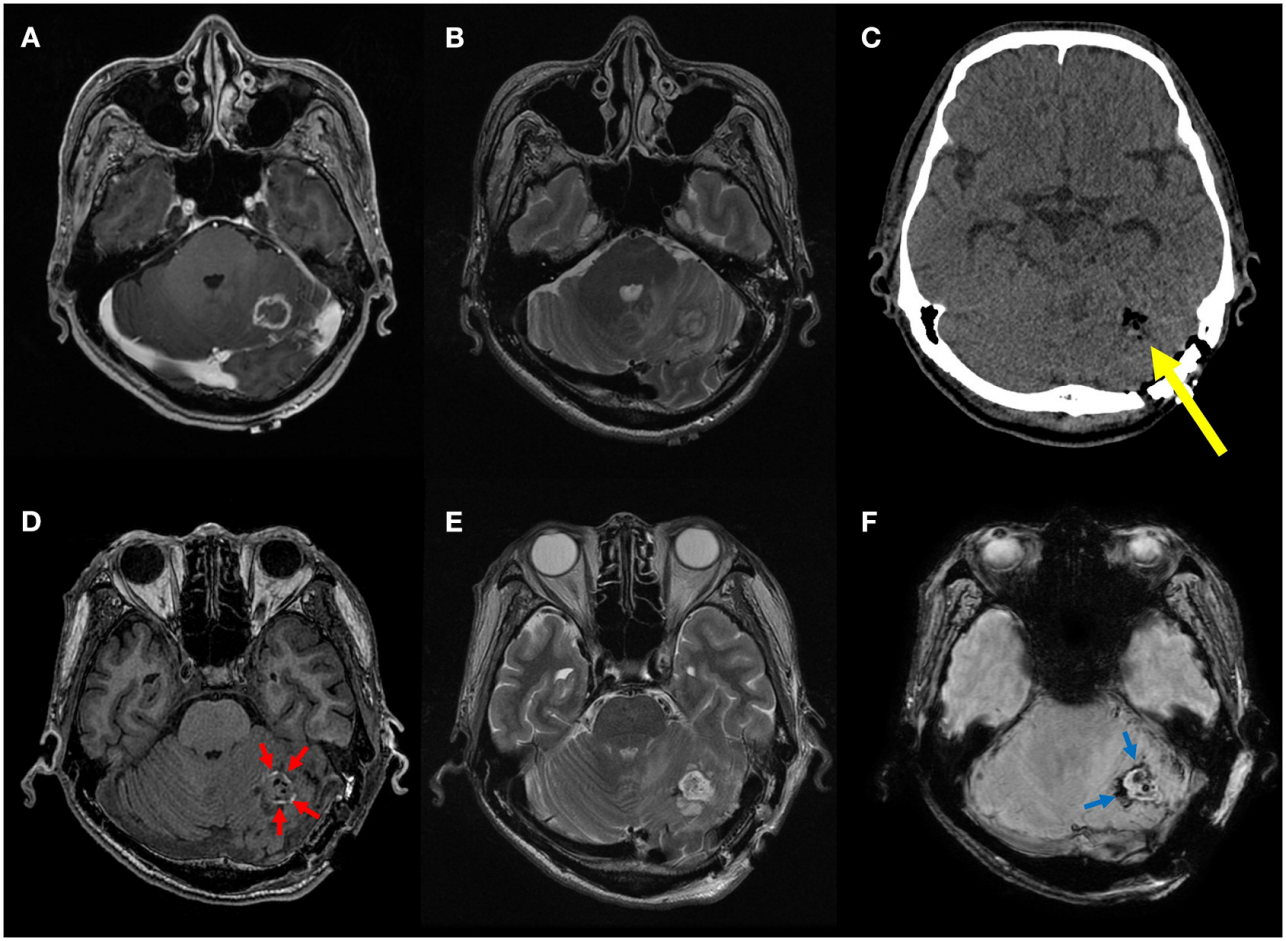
A representative case of minimal residual blood at the resection site. Preoperative T1 contrast-enhanced (A) and T2-weighted MR images (B) of a 62-year-old male patient with non-small cell lung cancer show a small rim-enhancing metastatic tumor in the left cerebellar hemisphere with peritumoral edema. On postoperative CT image (C), only air bubbles are seen in the resection cavity without discernible hemorrhage (yellow arrow). On postoperative MR images, only thin T1 hyperintense (red arrows) and T2* hypointense rim (blue arrows) is seen along the resection margin without space-occupying hematoma on T1-weighted (D), T2-weighted (E), and susceptibility-weighted images (F). This was considered absence of postoperative intracranial hemorrhage, and the patient did not experience postoperative delirium.
